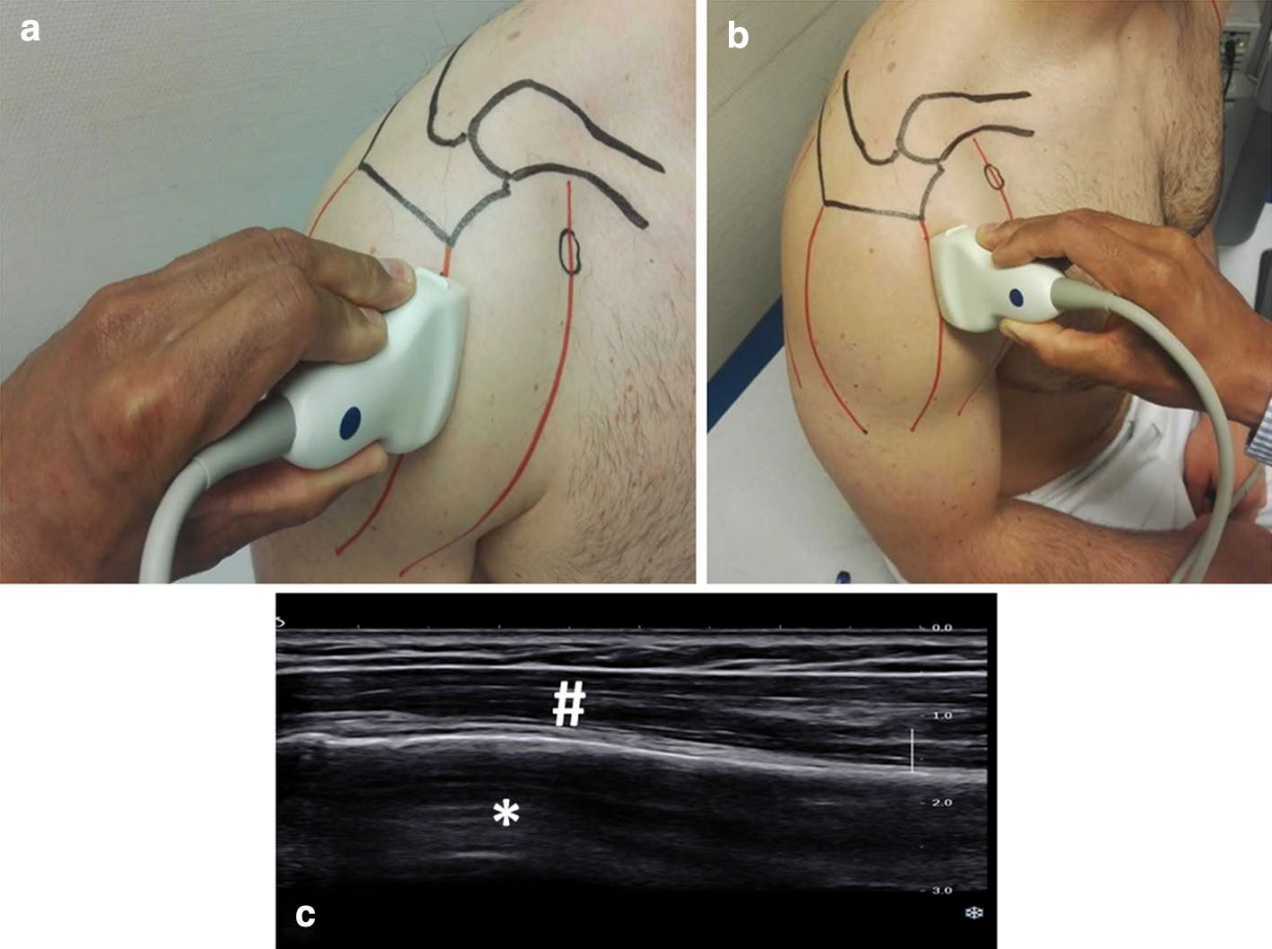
Examination set-up. Standardized examination set-up with the patient in a sitting position with the arm placed palm down on the patient’s thigh (a, b). Exemplary ultrasound image (c) showing the humeral neck (*) and the deltoid muscle (#)
